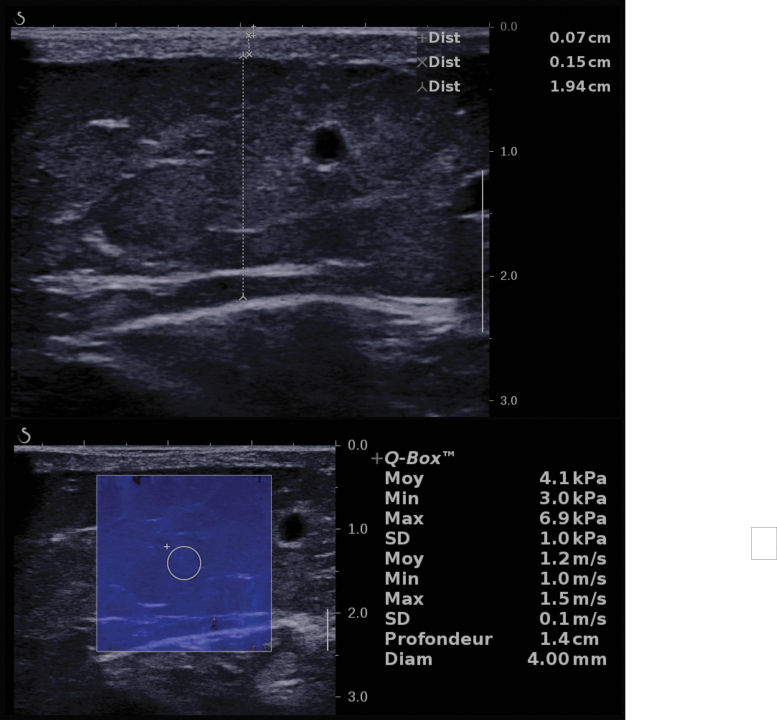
Ultrasonographic measurement of skin layer thickness and elastography. B-mode ultrasonographic image with thickness measurement of skin layers (*upper panel*), and elastography measurement (*lower panel*) in a limb with lymphedema.
